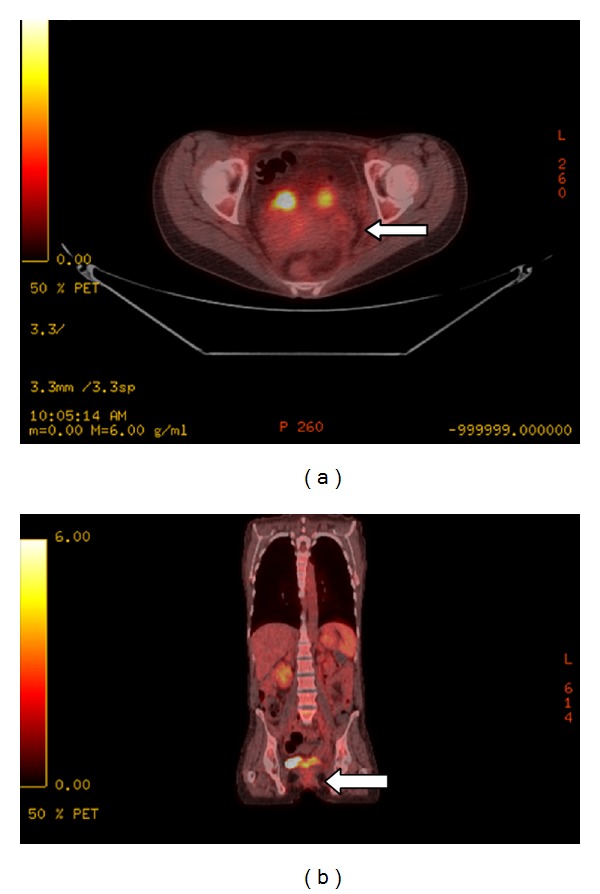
Axial (a) and coronal (b) PET-CT fusion images of the pelvis obtained a few months later after radiation demonstrate significant reduction in the lymphomatous mass (arrow), with no measurable SUV.
